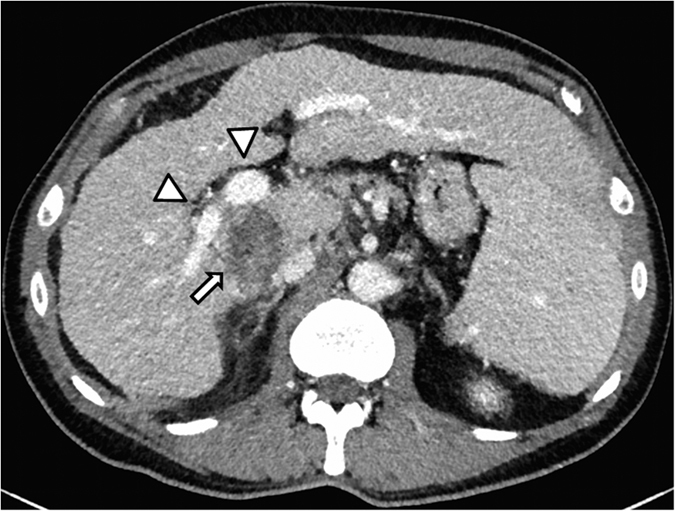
Same patient as in Figs 1, 2 and 3 with HCC. The post-interventional control CT the day after the intervention shows hypodense demarcation of the ablation defect (arrow), and the adjacent portal vein (tips of arrows) is thoroughly contrasted. No post-interventional complications.
